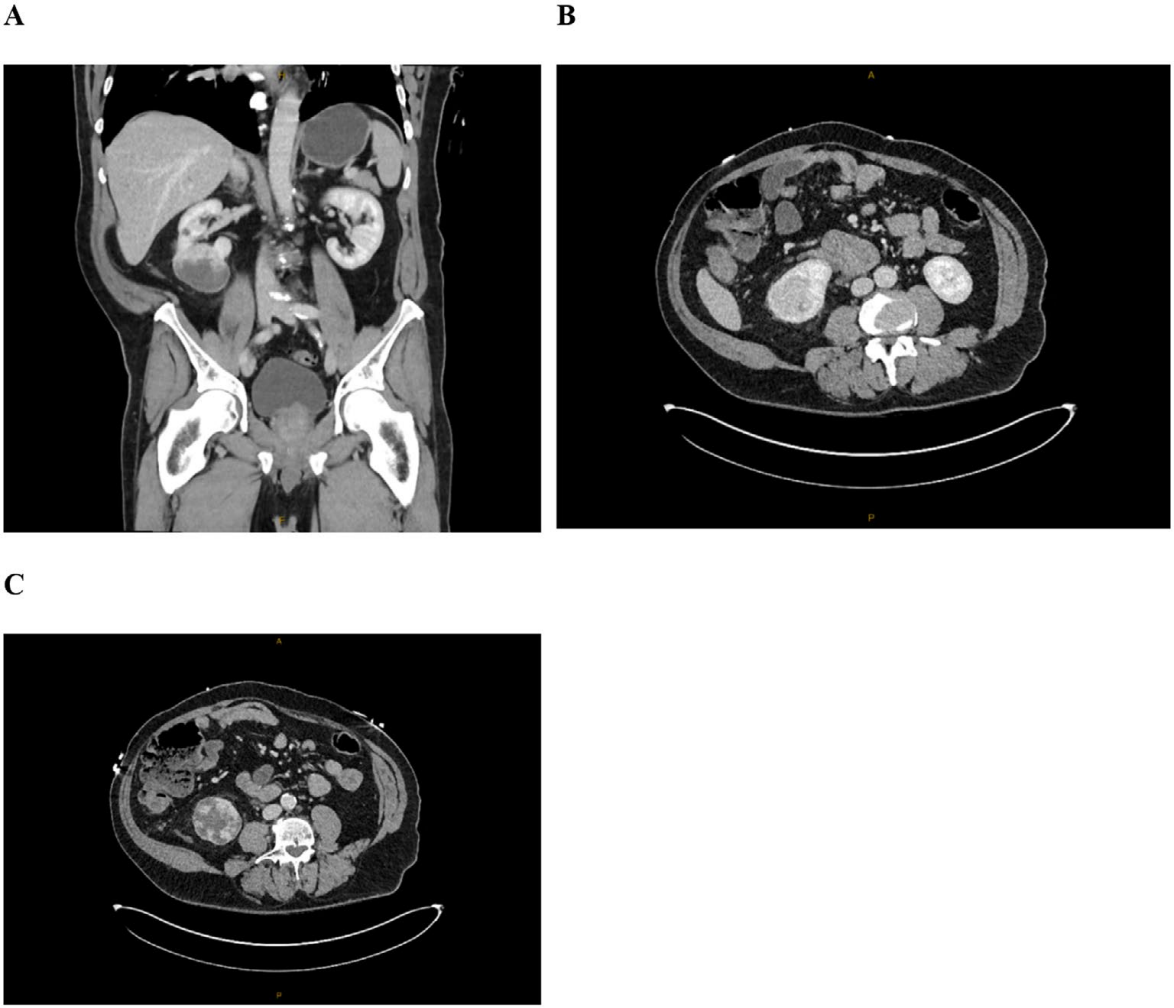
(A) Computed tomography scan of the abdomen and pelvis in the coronal plane indicating a 65 mm heterogeneous vascular mass that was identified arising from the lower pole of the right kidney. (B, C) Computed tomography scan of the abdomen and pelvis in the axial plane indicating a 65 mm heterogeneous vascular mass that was identified arising from the lower pole of the right kidney.
